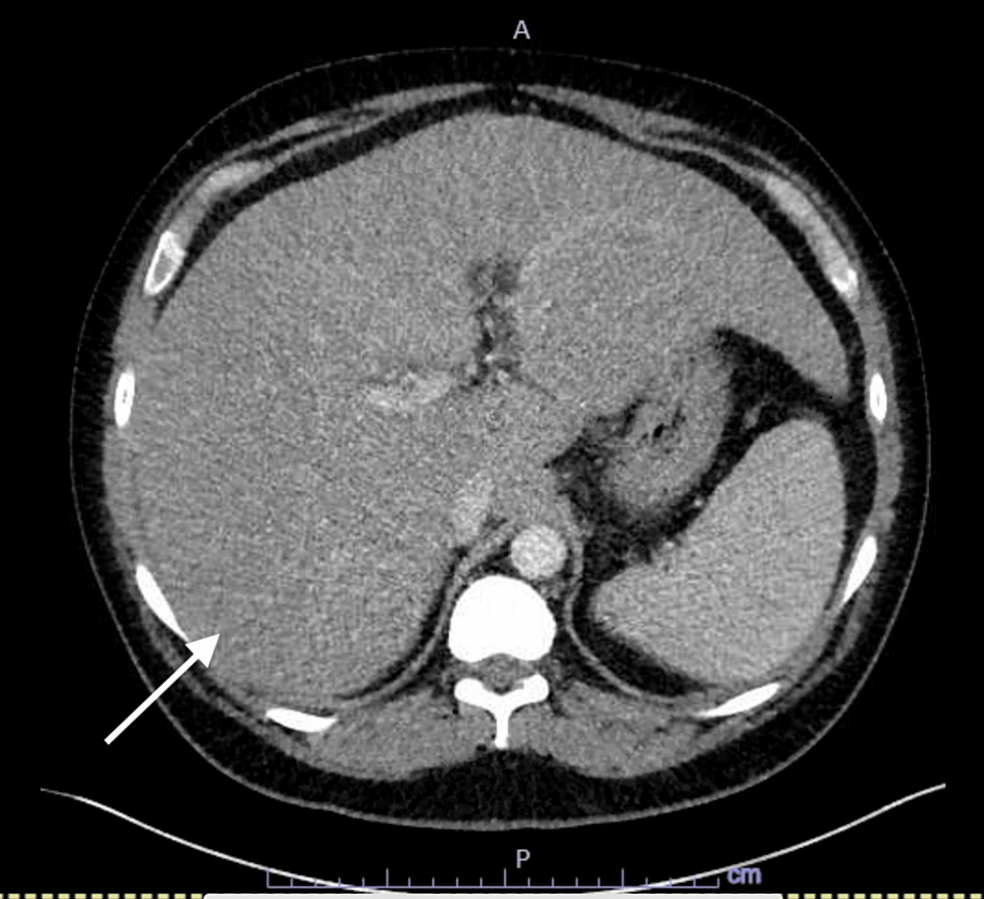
Cross-sectional computed tomography scan of the abdomen Cross-sectional abdominal computed tomography (CT) scan showing evidence of hepatomegaly (arrow).
